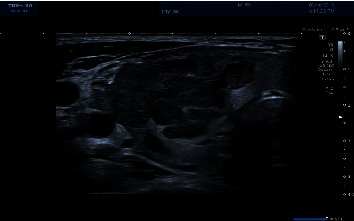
Thyroid ultrasound: linear probe and high frequency. Right lobe: hypoechoic polylobular nodule with intense intranodular vascularisation classified as EU-TIRADS 5.
